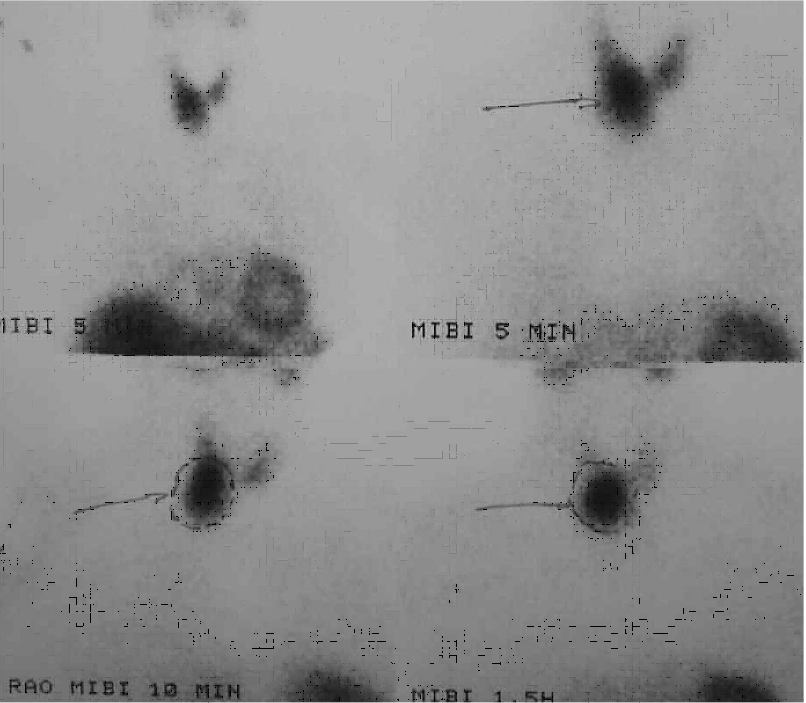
Imaging of the parathyroid paraesophageal adenoma by Tc-99m scintigraphy
